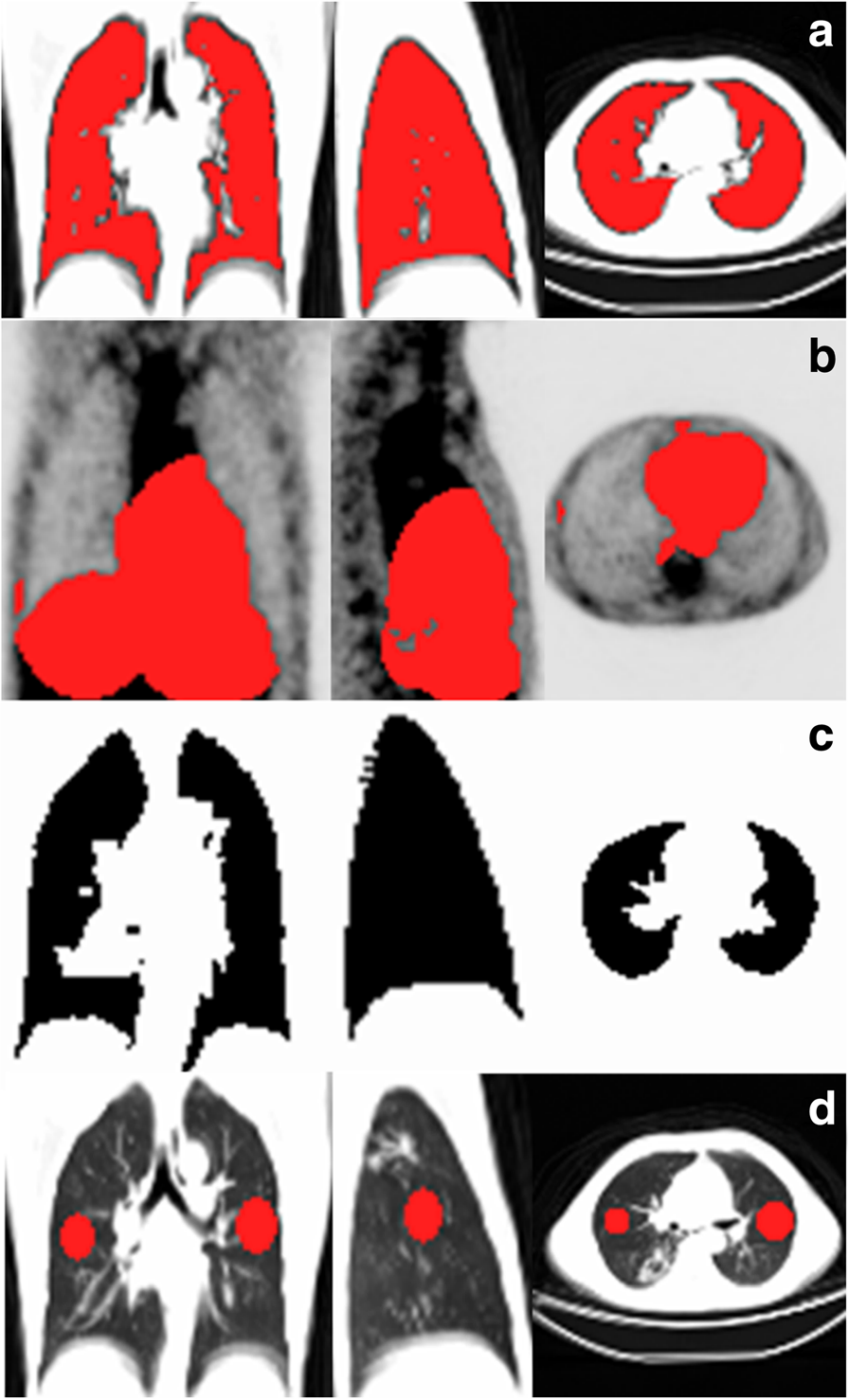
Example of volumes of interest (in red) required for quantification. a Lung mask, on the combined CT images from baseline and follow-up scans. b Volume of structures surrounding the lung affecting motion misregistration (usually the mediastinum, liver and spleen) drawn on the overlaid PET images. We then deleted overlapping areas on the lung mask to leave only areas unaffected by misregistration. c Binary image representing the final lung mask. d Reference volumes of normal lung tissue, represented by two spheres in opposite lungs or ipsilateral lobes. It is also drawn on the overlaid CT images, but viewed alongside the PET images to ensure that it represents lesion-free lung
